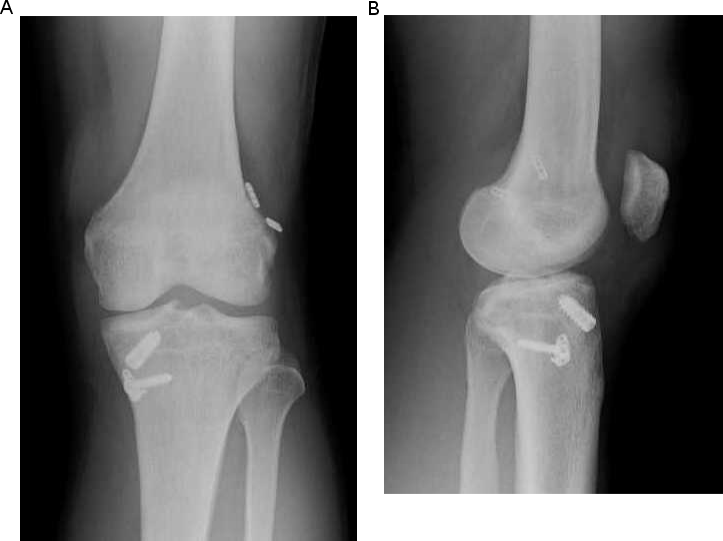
A and B Postoperative radiograph. A: anterior to posterior view, B: lateral view.
